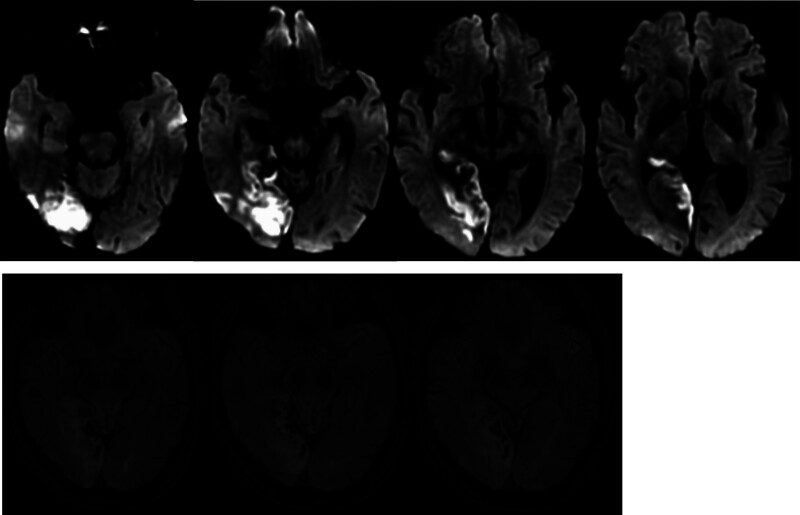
(A) DWI sequence shows hyperintensity in the right temporo-occipital lobe. (B) SWI sequence indicates abnormal signals in the right temporo-occipital lobe, so multiple microhemorrhagic lesions are suspected. DWI = diffusion weighted imaging, SWI = susceptibility weighted imaging.
